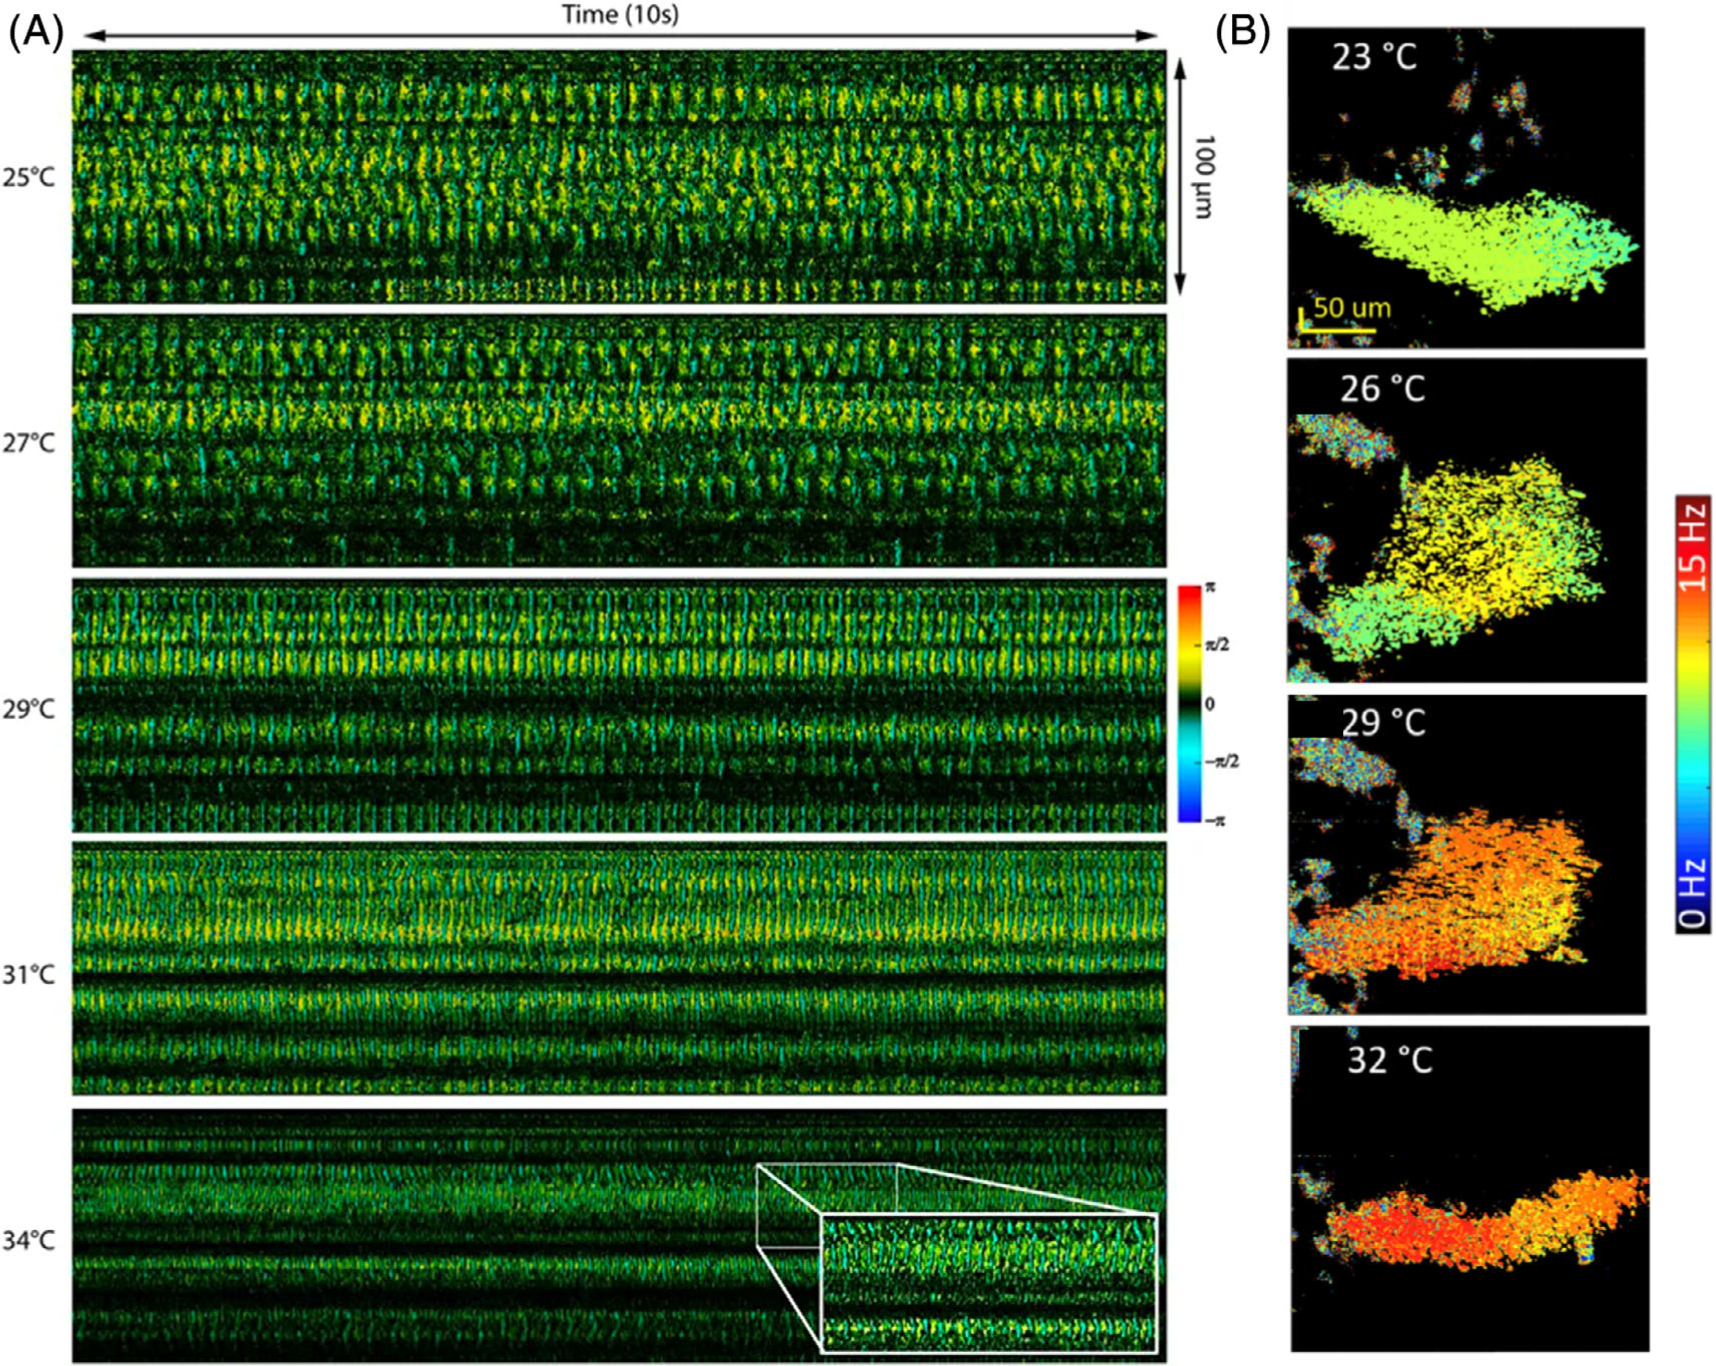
(A) Doppler images of ciliary motion at temperatures of 25°C, 27°C, 29°C, 31°C, and 34°C, in which cilia beating frequency under different temperatures were observed. (B) Spatial distribution of the cilia beating frequency at 23°C, 26°C, 29°C, and 33°C in which temperature has a positive impact on ciliary activity. Adapted from References [86, 87]
